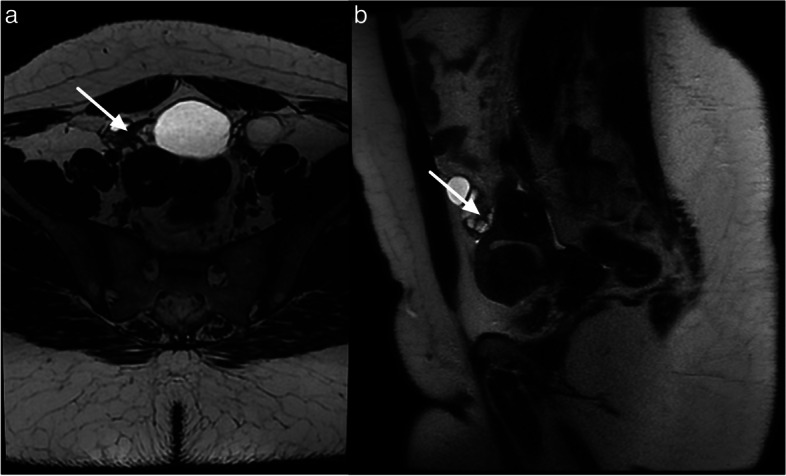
Fimbrial end of the tube between a dilated hydrosalpynx and the right ovarian structure. a Axial T2-weighted sequence. b Sagittal T2-weighted sequence. Stellar morphology of the fimbrial end of the tube (white arrow)Smooth wall or septa (Fig. 13) as opposed to irregular wall or septa.
